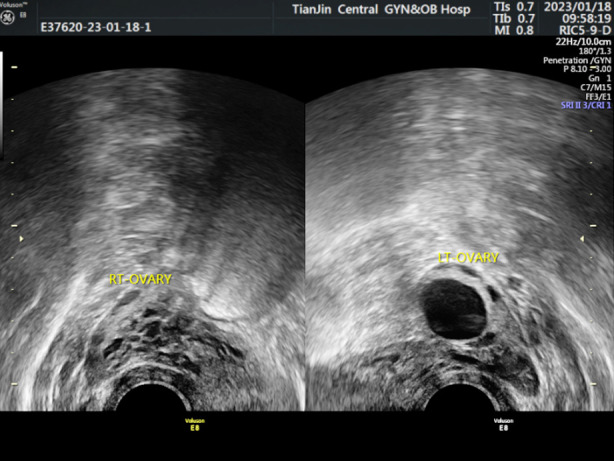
Ultrasonic examination image of Normal-group.
